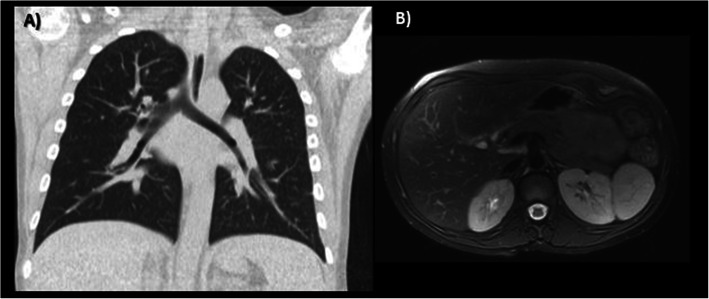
Complete resolution of pulmonary and abdominal viscera infiltrates is seen in A) Coronal non-enhanced lung CT, and B) Axial FS T2WI MRI
